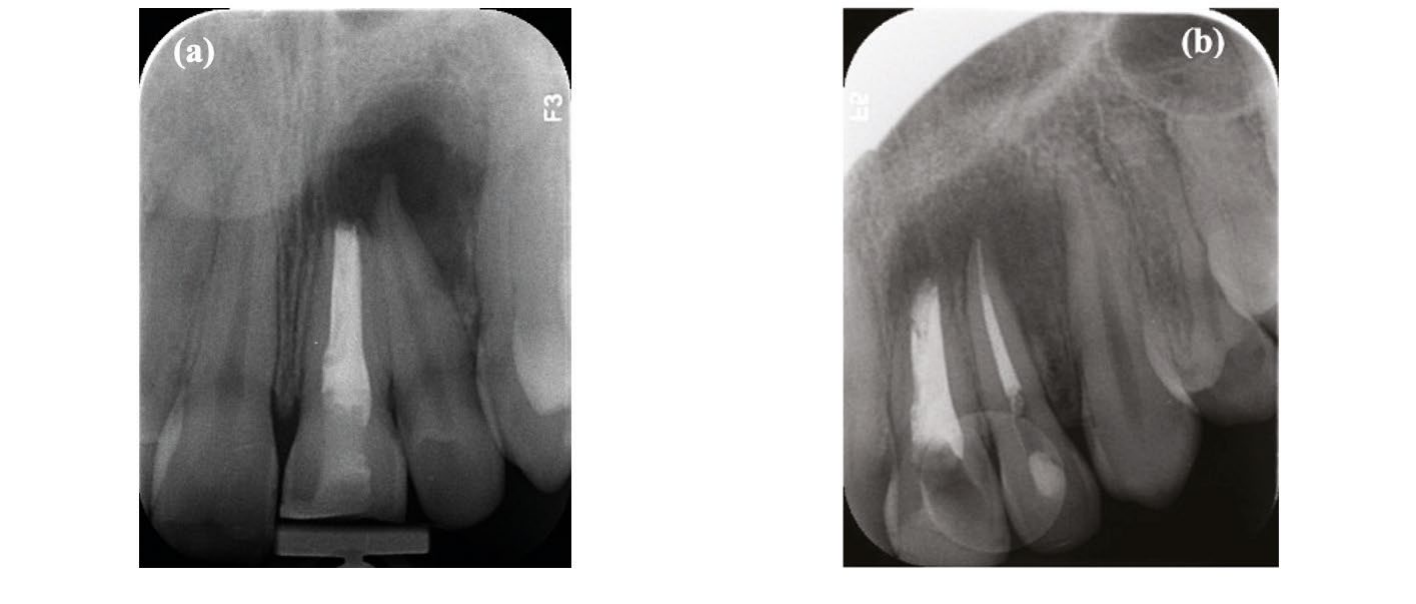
a) At the consultation visit, the patient complained of a discoloured tooth but had no pain. A pre-operative radiograph was taken as routine examination before commencing bleaching which shows a lesion, highlighting the importance of radiographic examination for diagnosis. b) A post-operative radiograph after re-treatment of the left maxillary central incisor and root canal treatment of the left maxillary lateral incisor
